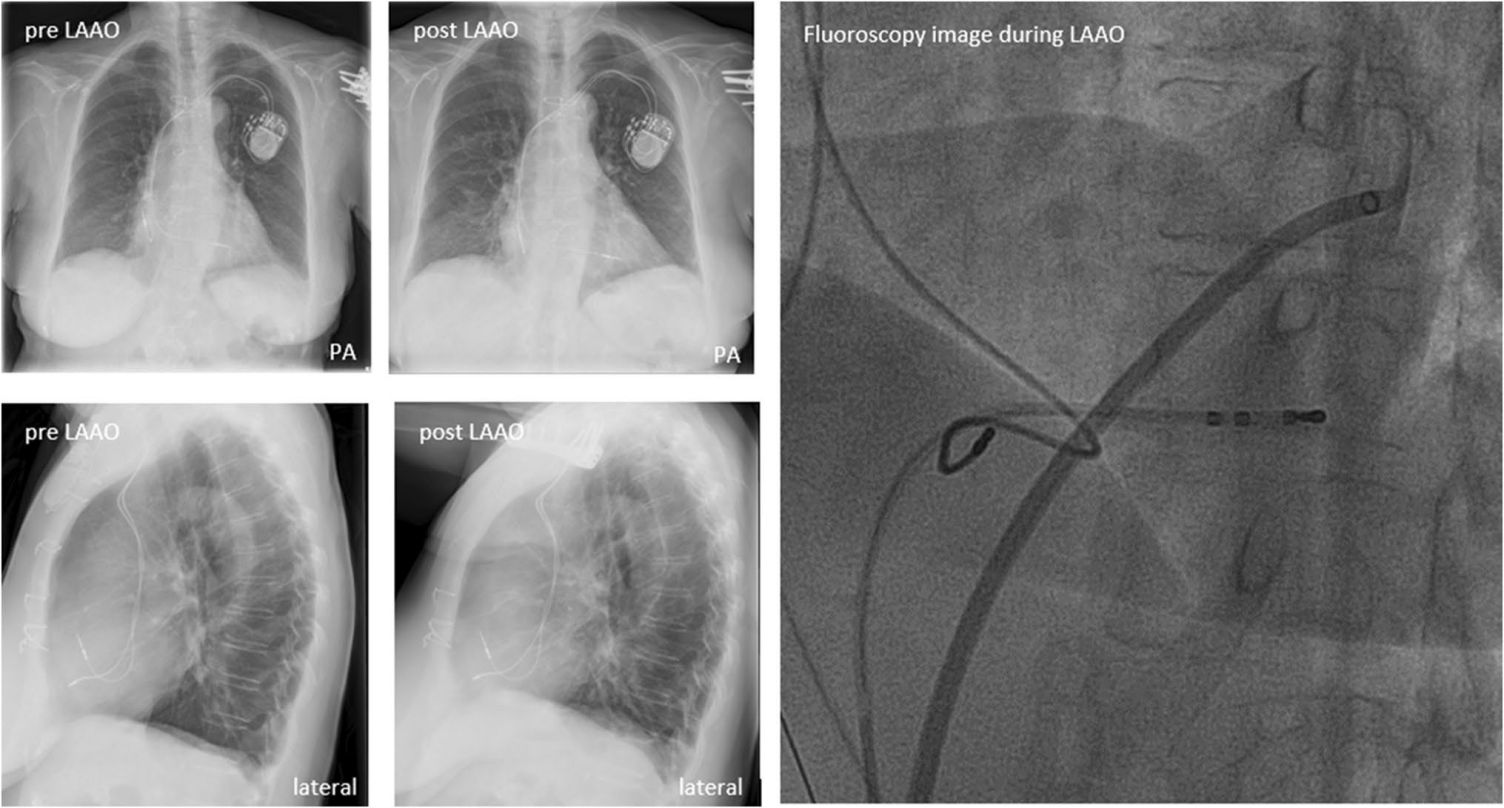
Typical examples patient with CIED and TVL. (Left) Chest X-rays posterior–anterior view and lateral view, prior, and post LAAO with CIED and TVL in situ. (Right) Fluoroscopy imaging showing TVL and access sheath percutaneous LAAO. CIED: cardiac implantable electronic device; LAAO: left atrial appendage occlusion; PA: posterior–anterior; TVL: transvenous leads
